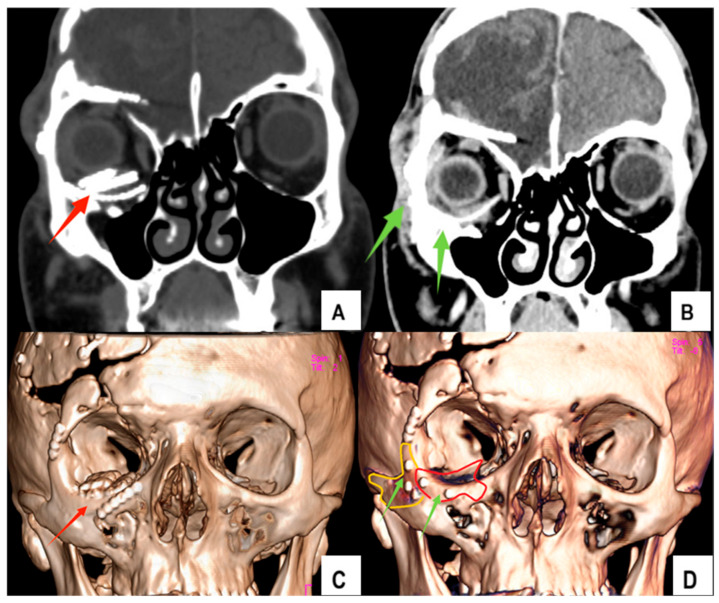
Preoperative CT and 3D reconstruction showing malpositioned multiple titanium plates (A,C); (red arrows). Postoperative CT and 3D reconstruction at 6 months demonstrating personalized PEEK and PDMS implants in the planned position (B,D); (green arrows). PEEK implant projection is highlighted in red (D) and PDMS projection in orange (D).
